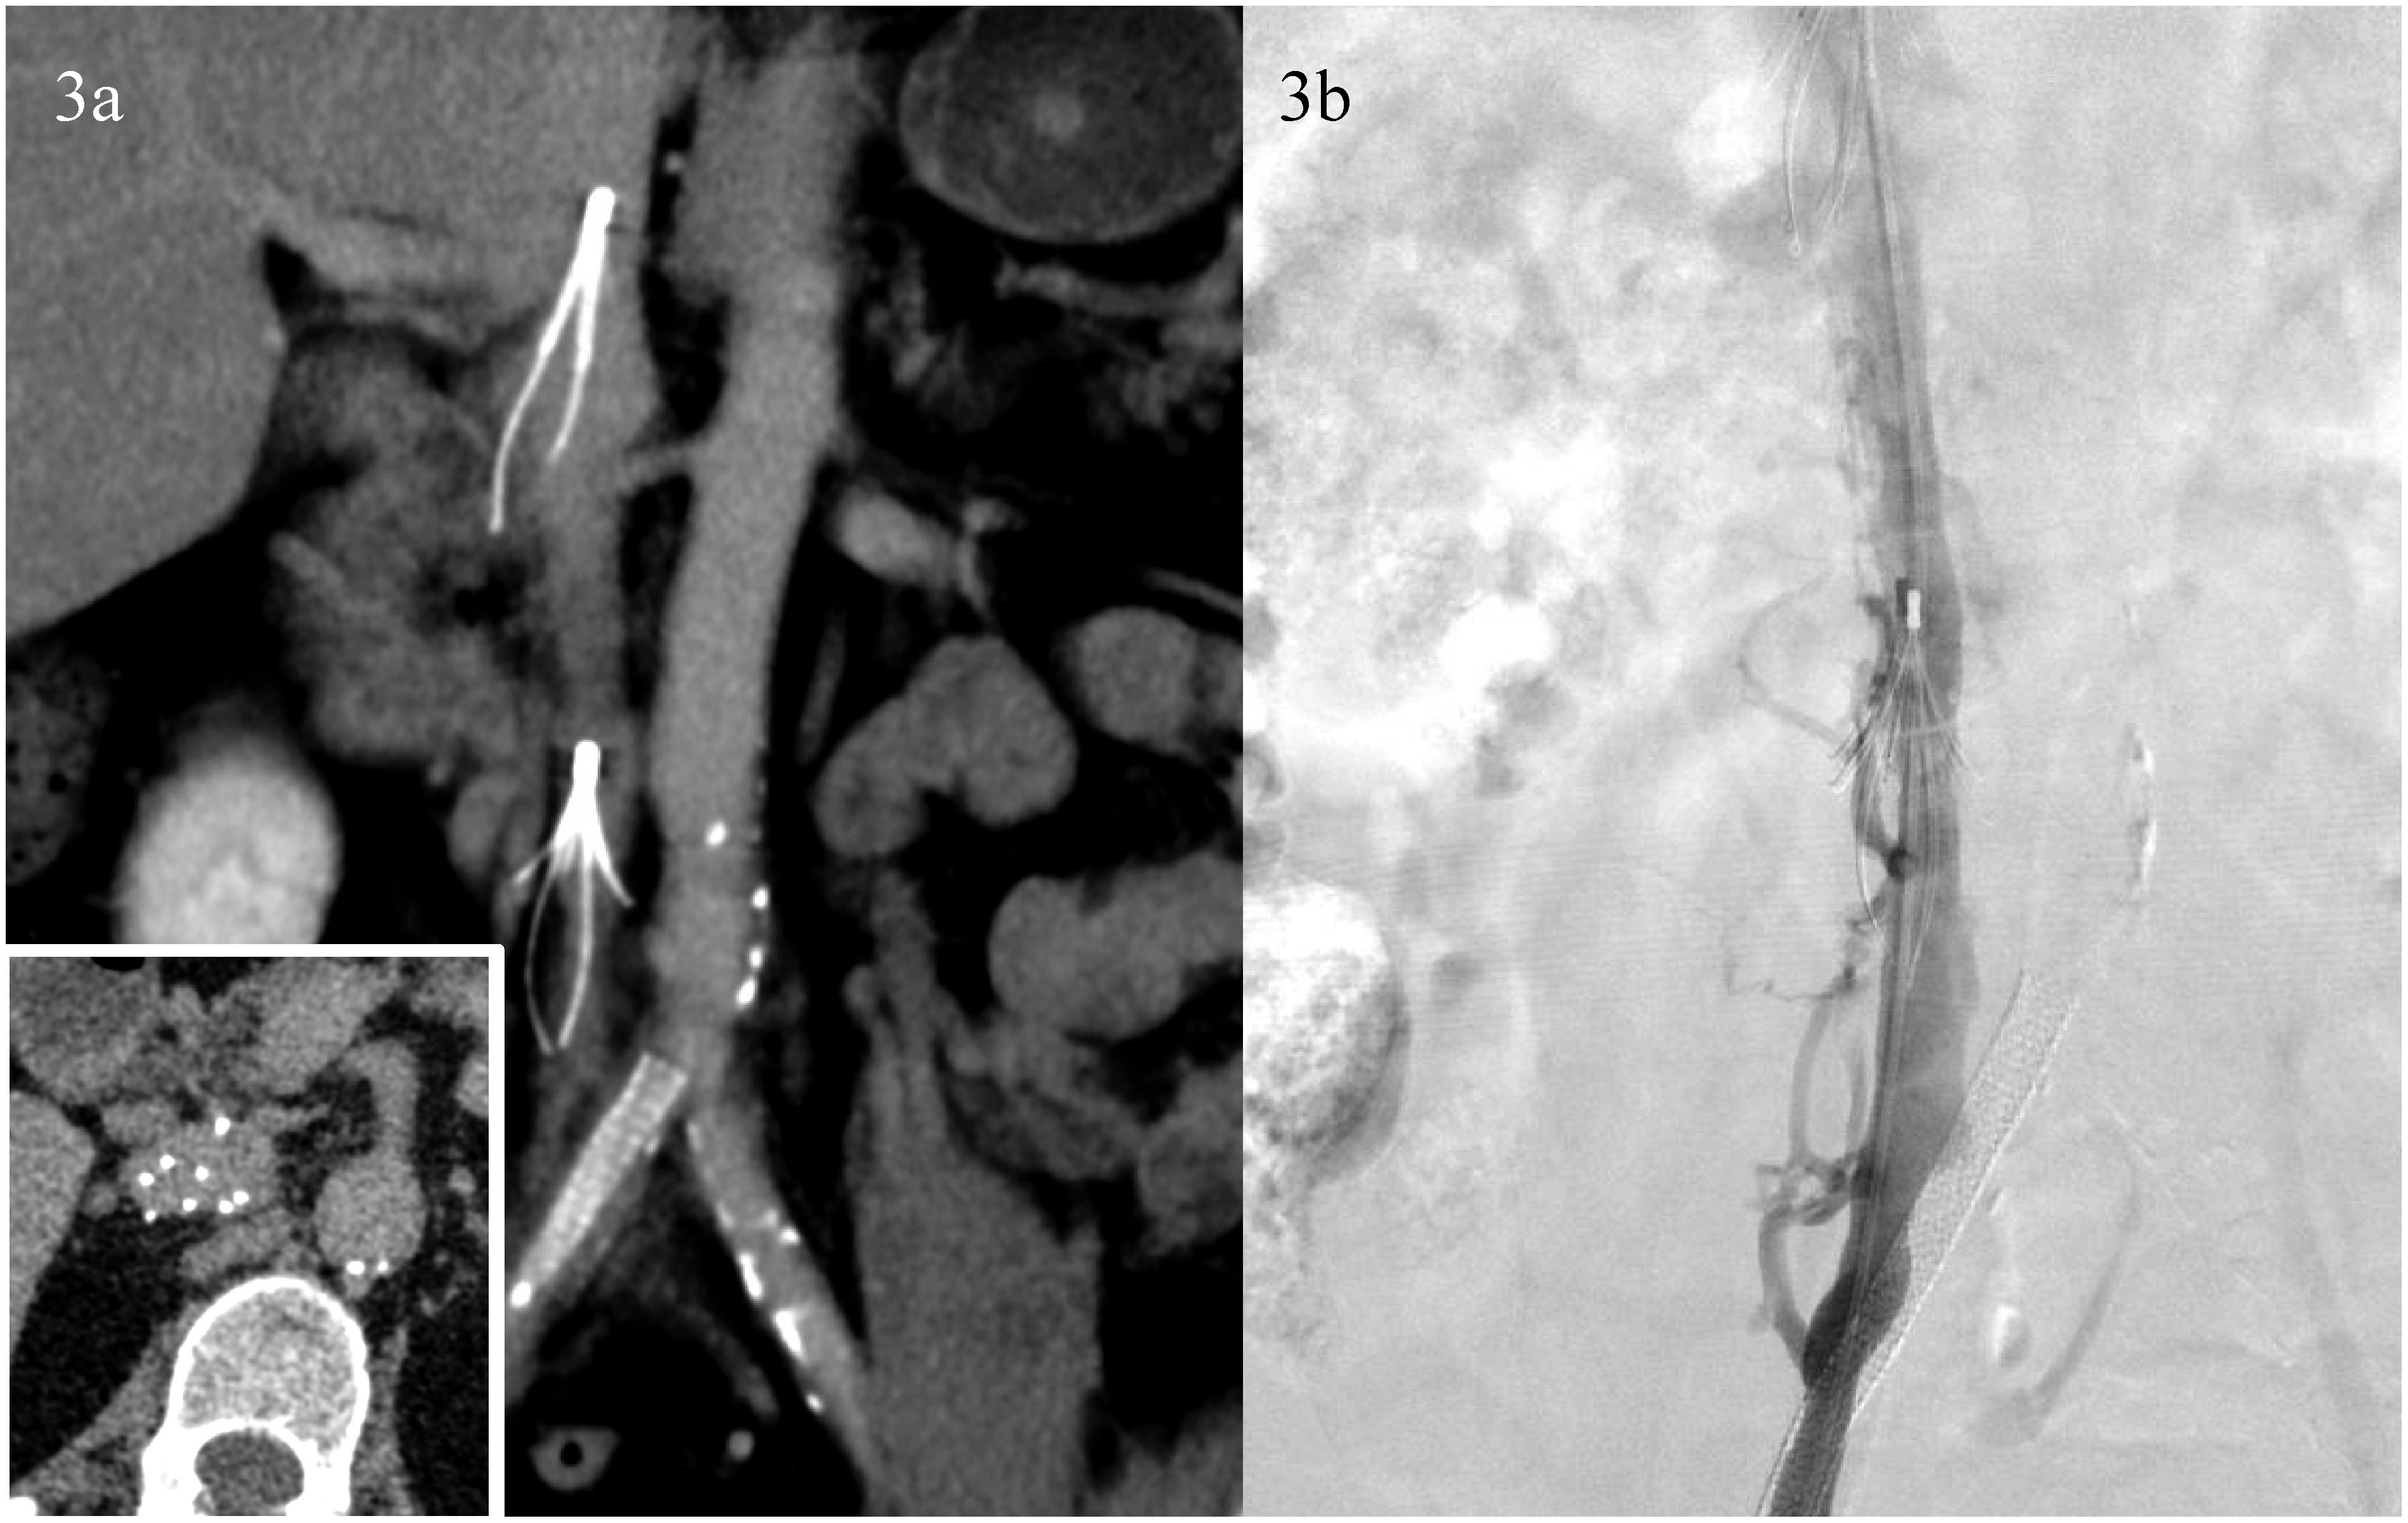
Fig. 3 Computed tomography (a) and angiogram (b) taken prior to inferior vena cava (IVC) filter retrieval show no thrombus in the IVC. Axial computed tomography image (a, lower left corner) demonstrates proximal filter penetration. However, no organs or the structure are involved.
